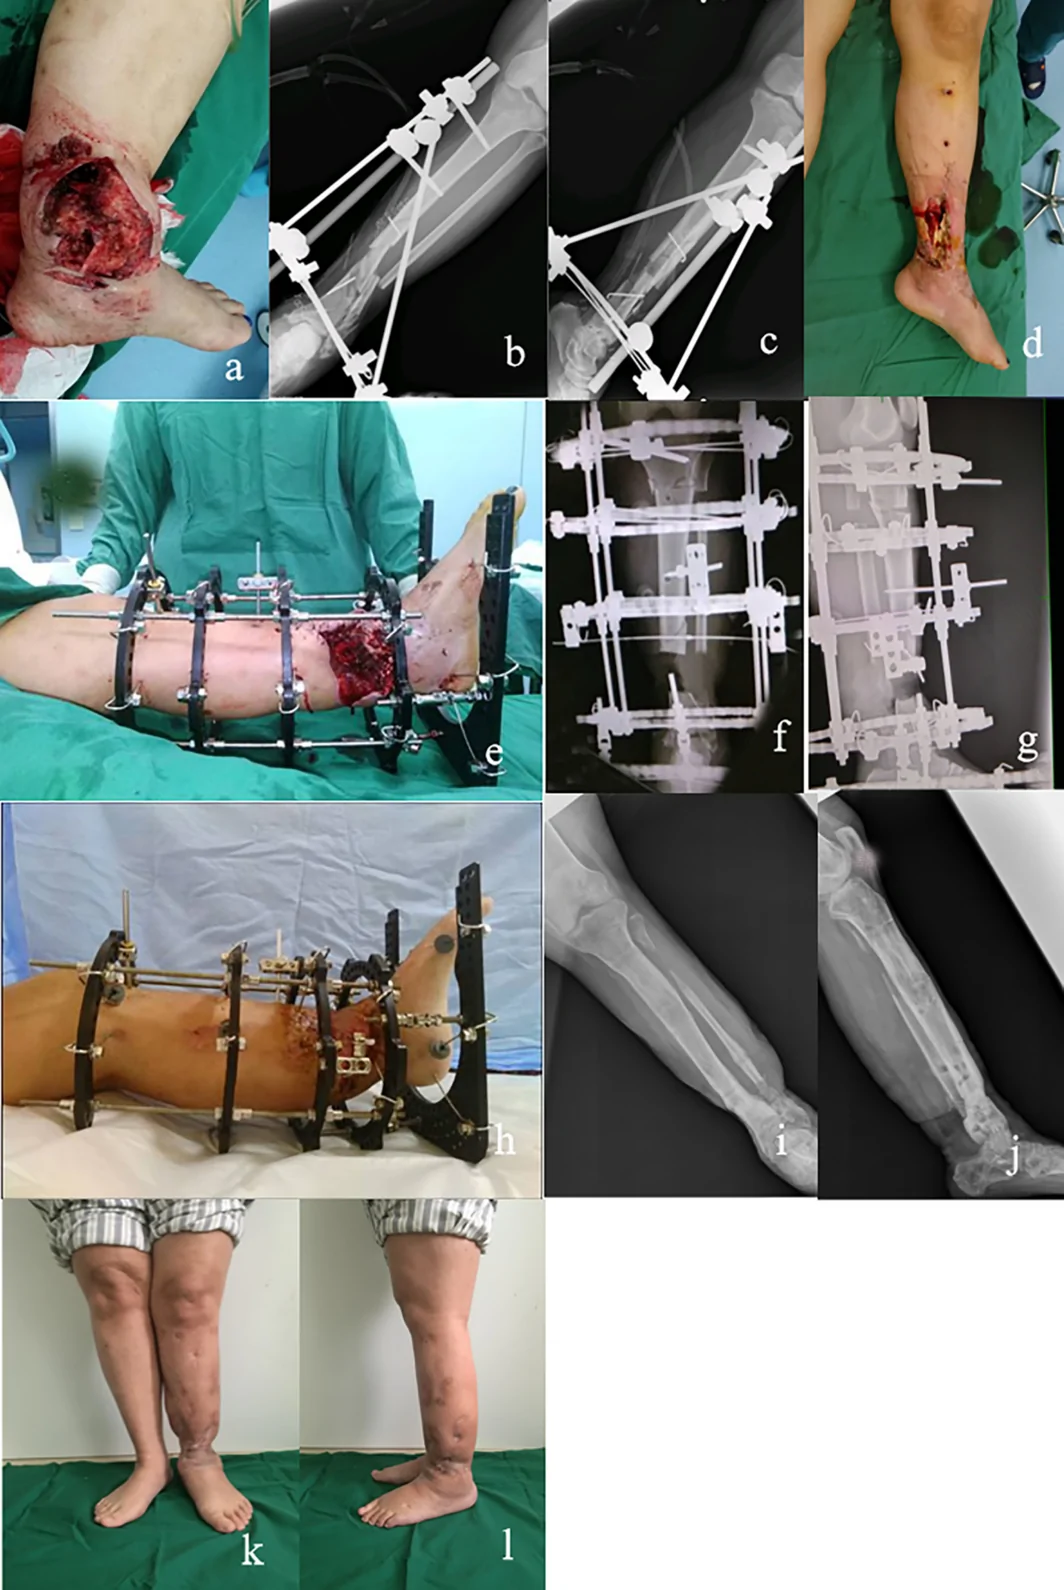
A 45‐year‐old female with ITBD‐STD treated with the S‐L technique. (a) Clinical photograph of the left lower leg at the time of injury. (b, c) Radiographs showing a comminuted tibial fracture fixed with a hybrid external fixator. (d) Clinical photograph demonstrating tibial exposure with an associated soft tissue defect. (e) Intraoperative photograph following debridement of the distal left lower leg, showing bone and soft tissue defects stabilized with a circular external fixator. (f, g) Postoperative radiographs showing resection of the infected middle and lower tibial segment with partial shortening, stabilized with a circular external fixator, and proximal tibial osteotomy. (h) Completion of left lower leg shortening‐lengthening with circular external fixation. (i, j) Postoperative radiographs demonstrating external fixator removal, adequate mineralization at the lengthening site, and solid union at the docking site. (k, l) Clinical follow‐up photographs taken 1 month after removal of the circular external fixator.
